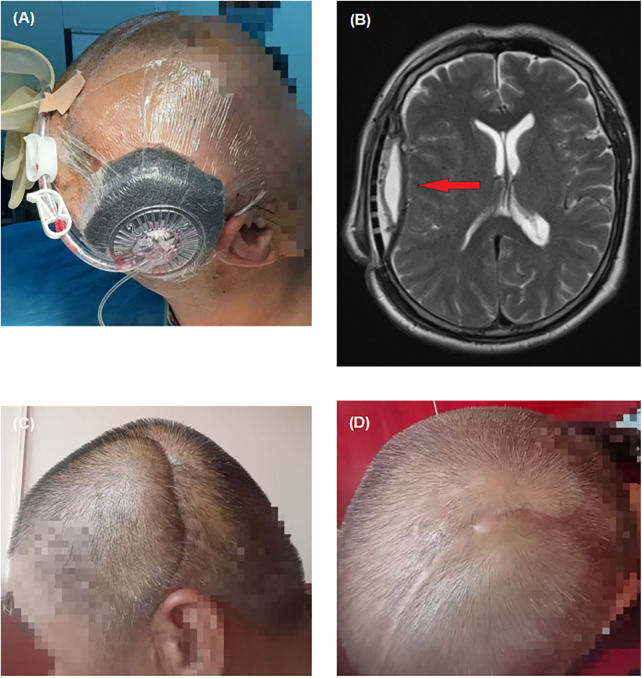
Vacuum sealing drainage application and follow-up outcomes. (A) Clinical photograph demonstrating vacuum sealing drainage (VSD) device placement. (B) Magnetic resonance imaging (MRI) showing resolution of scalp soft tissue swelling and inflammatory hyperintensity in the previously infected region, with uniform signal intensity across all layers and controlled acute inflammation; small epidural fluid collection in the right frontotemporal region, normal signal in subgaleal and other spaces without fluid or pus accumulation, suggesting cleared infection. (C,D) Three-month follow-up clinical photographs in sagittal and axial views demonstrating scalp recovery: no significant erythema, exudate, or dehiscence, with no evident infectious inflammation at the incision site.
